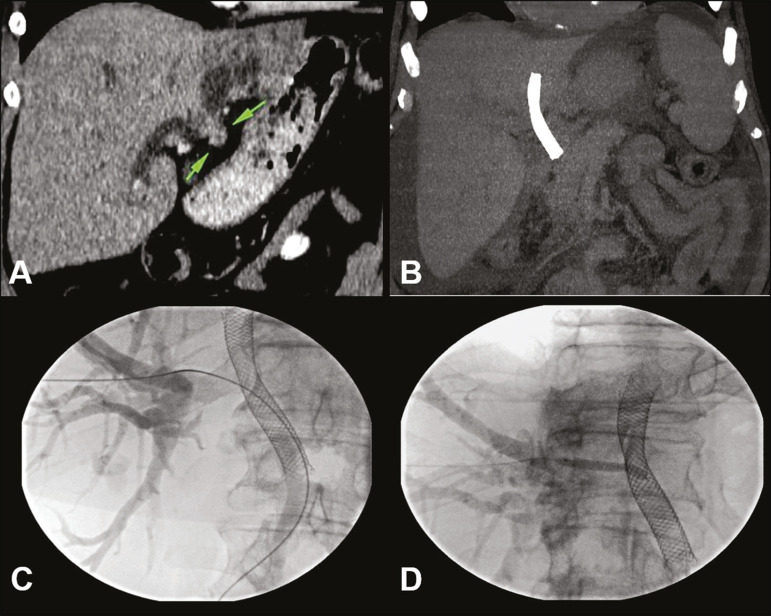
A 71-year-old male patient presenting with obstructive jaundice due to neoplasia (A). A coated, covered metal biliary stent was placed via ERCP. However, the stent placement did not result in an improvement in the obstruction. Subsequently, ERCP was again performed in order to place another (fenestrated) stent within the previously positioned stent. Computed tomography showed the original stent positioned in the left biliary tract (atrophied hepatic segment) and increased biliary dilatation in the right hepatic lobe (B). Because of the cholangitis, external percutaneous drainage of the right biliary tract was performed as an emergency procedure. After 60 days, the patient underwent the removal of the external drain and the RUPS-100 kit was used in order to introduce a hydrophilic wire into the original stent (C). Direct percutaneous puncture of the coated biliary stent was performed with the RUPS-100 cutting needle, after which a 5 × 40 mm expanding coronary balloon stent was positioned (D). The procedure resulting in technical success with no complications.
